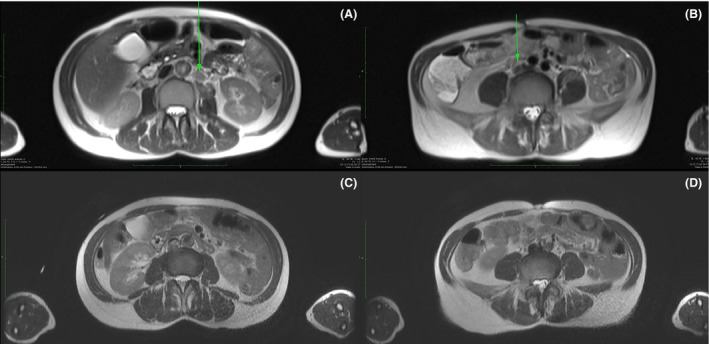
Follow‐up Magnetic Resonance Images (MRI) of the patient after IRECT. A and B. 24 h post IRECT, showing no signs of bleeding, hematoma, fluid or air collections in the two treatment areas. C and D 8 months post IRECT, the treated lymph nodes show no signs of malignancy.
